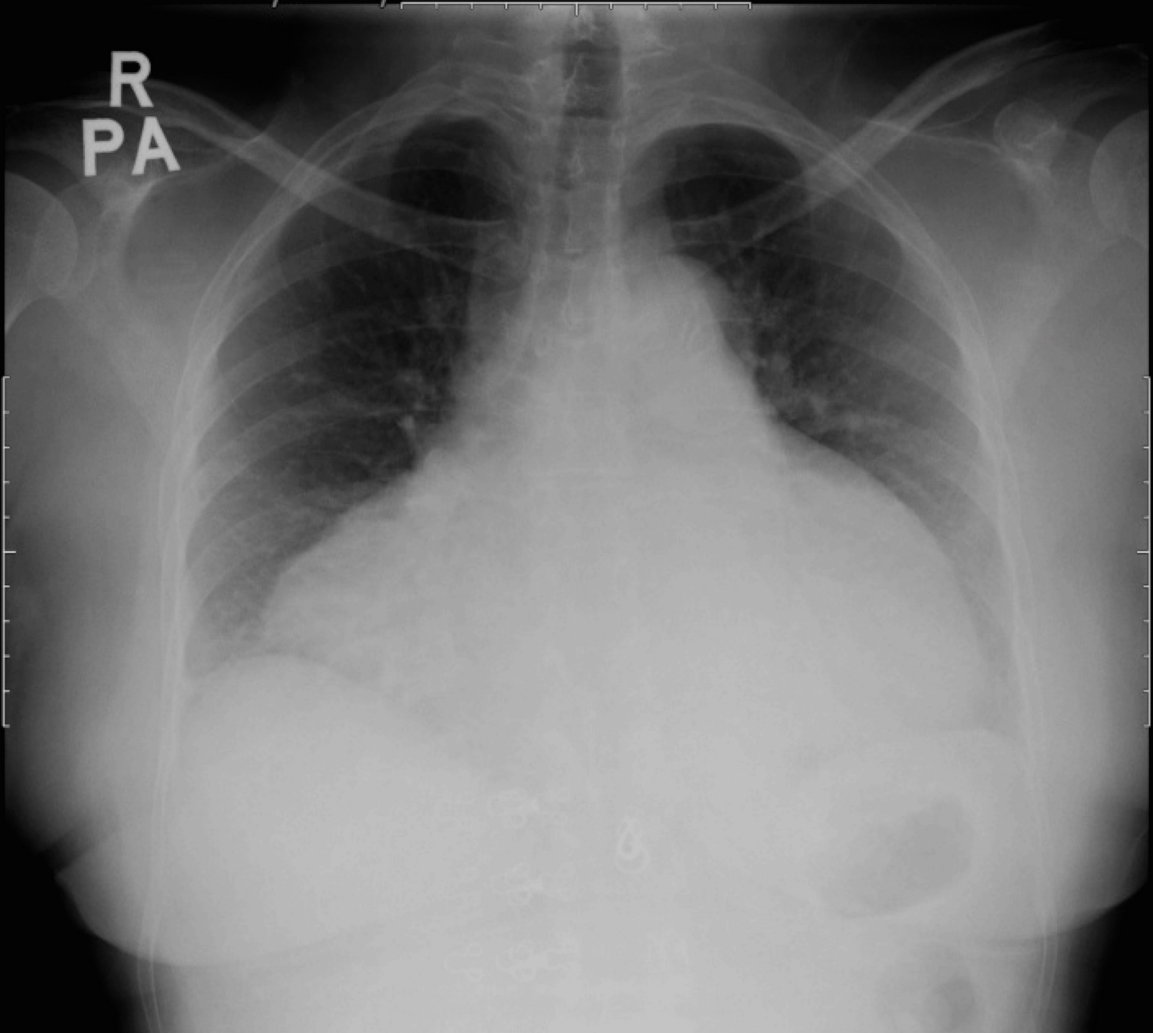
CXR on initial presentation demonstrating globular enlargement of the cardiac silhouette, resembling a water bottle configuration CXR: chest X-ray
